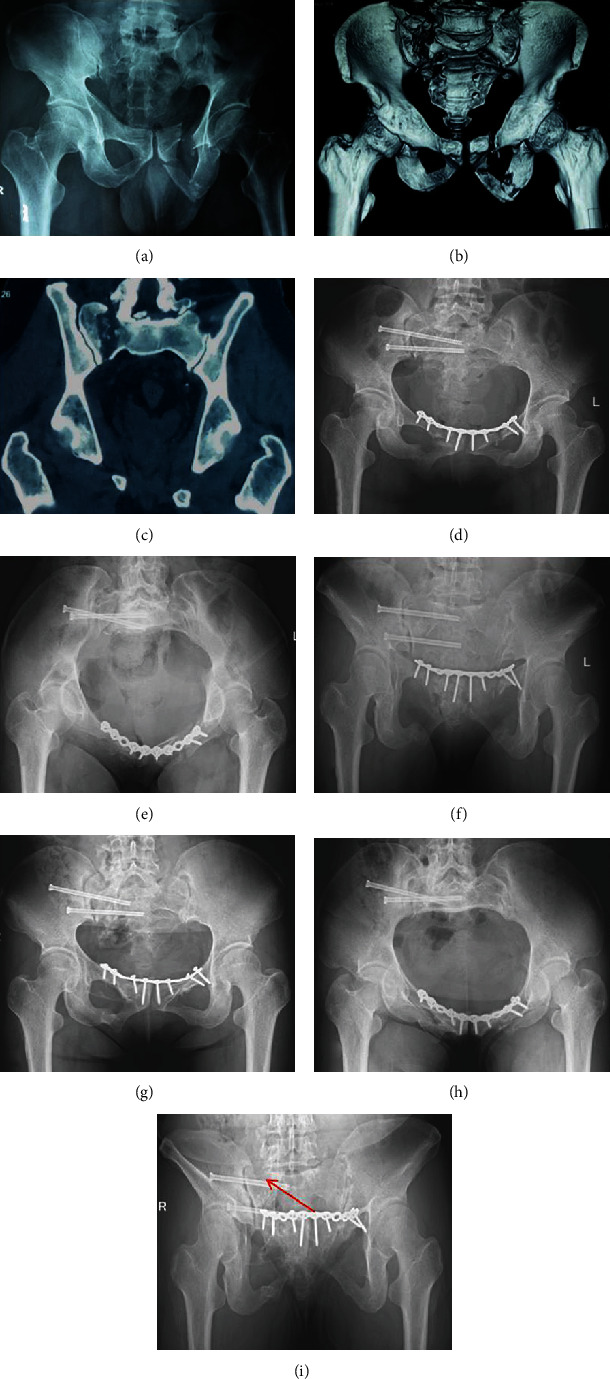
Patient, female, 46, pelvic fractures, front ring plate fixation. The posterior ring was fixed with double sacroiliac screws. (a) Anteroposterior view of pelvic fracture. (b) 3D CT preoperative pelvic fractures for Tile C1.3 type, unilateral vertically unstable pelvic ring. (c) Preoperative CT scan in pelvic posterior ring by the sacrum fracture, vertically displaced. (d) Anteroposterior radiograph of the pelvis immediately after the operation showed satisfactory reduction of the fracture. (e) Inlet radiograph of the pelvis immediately after surgery. (f) Outlet radiograph of the pelvis immediately after surgery. (g) Anteroposterior radiographs of the pelvis 20 months after surgery showed fracture healing. (h) A radiograph of the pelvic inlet 20 months after surgery showed fracture healing. (i) A radiograph at the exit of the pelvis 20 months postoperatively showed healing of the fracture and no loss of reduction at the arrow.
